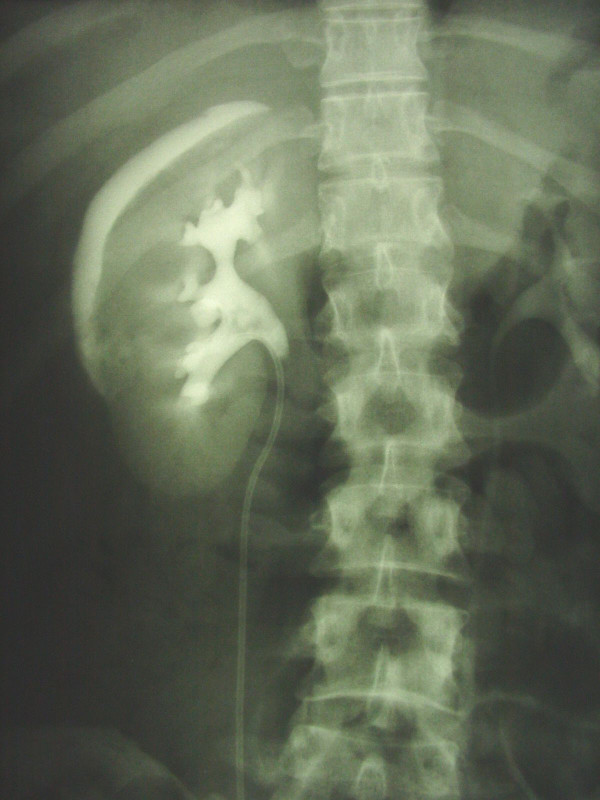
Abdominal plain radiograph with sickle-shaped perirenal contrast medium extravasation.
